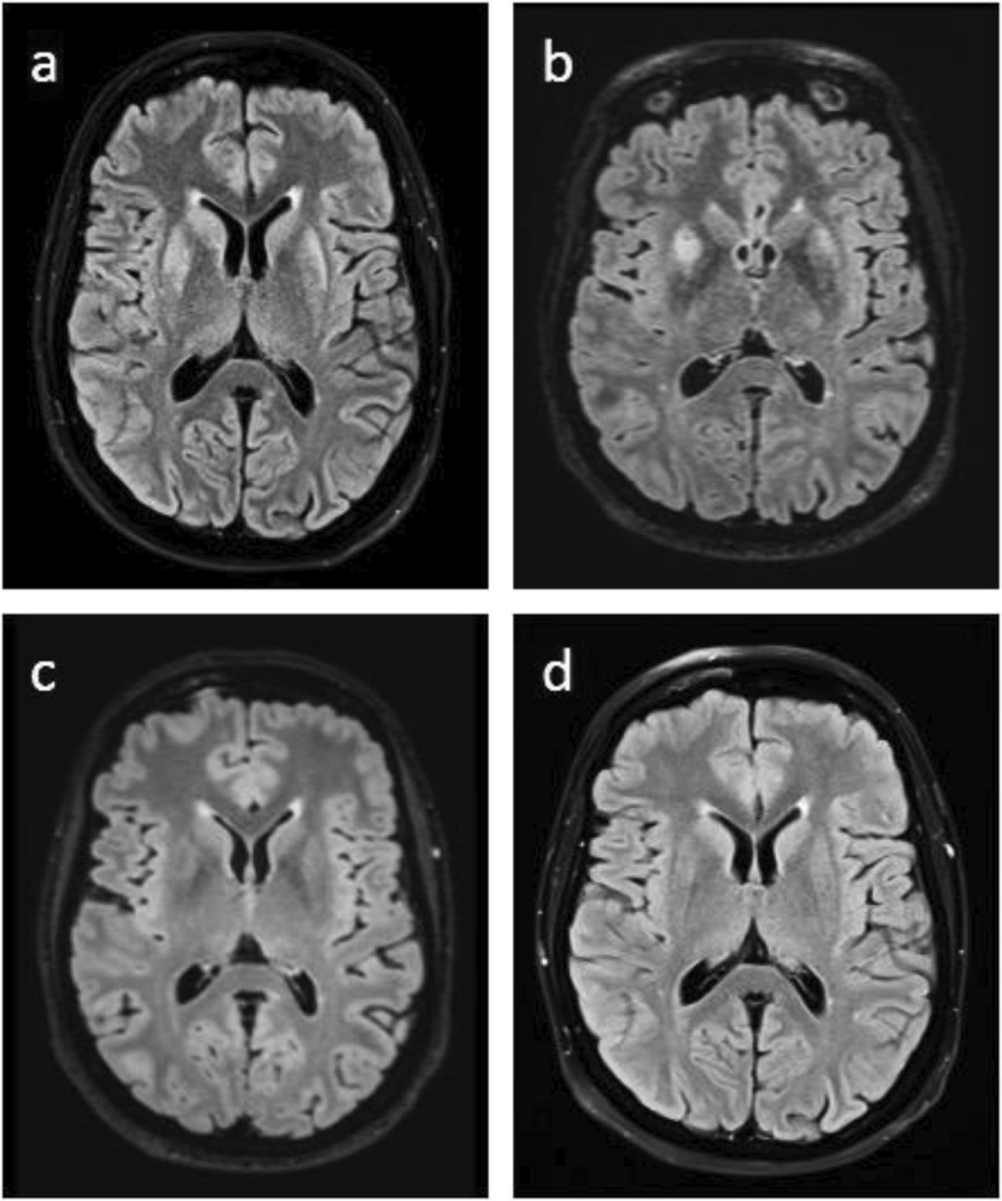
MRI T2-FLAIR imaging studies during the course of HHV-6 encephalitis. a Slight bilateral asymmetrical diffusion restricted areas in corpora striata. b Marked bilateral asymmetrical diffusion restricted areas in corpora striata. c Regression of diffusion restricted areas in corpora striata. d Normal appearance of corpora striata without evidence of diffusion restriction
